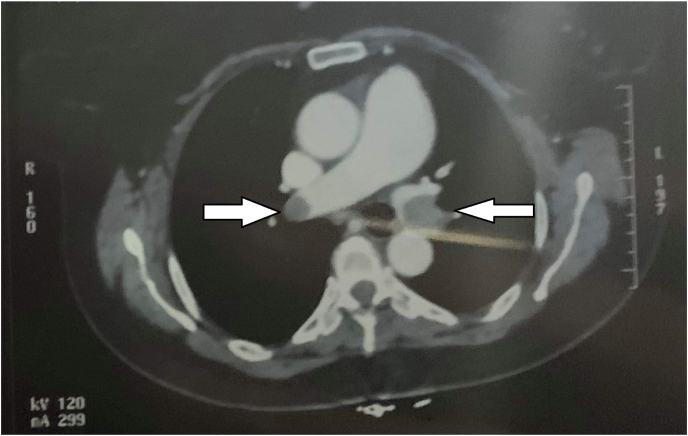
Thoracic computed tomography before any treatment. the two main pulmonary artery are enlarged.
